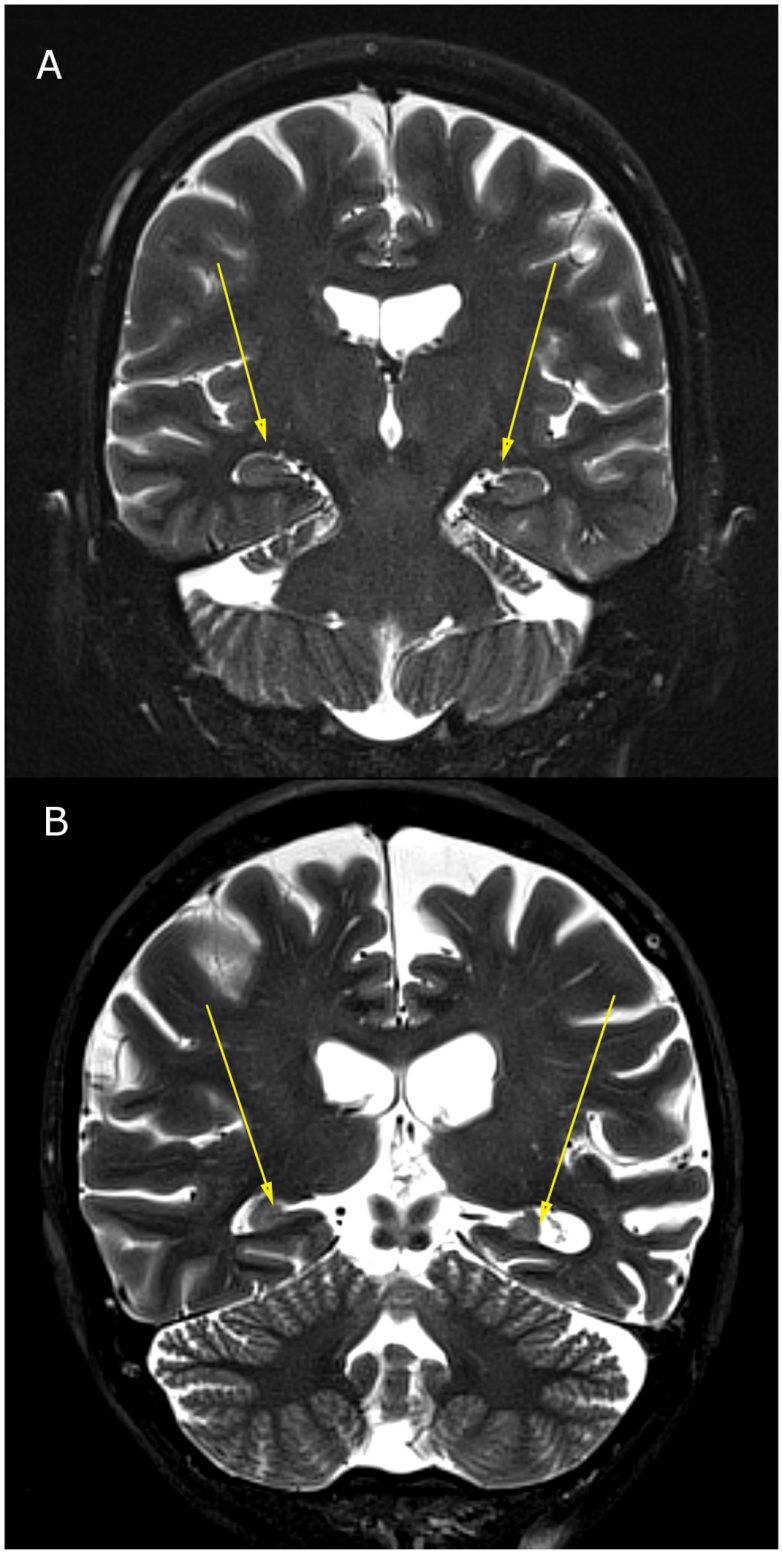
3T MRI of hippocampal atrophy; (1a) Normal hippocampal formations in 60-year-old female; (1b) Severe hippocampal atrophy in 60-year-old female.
